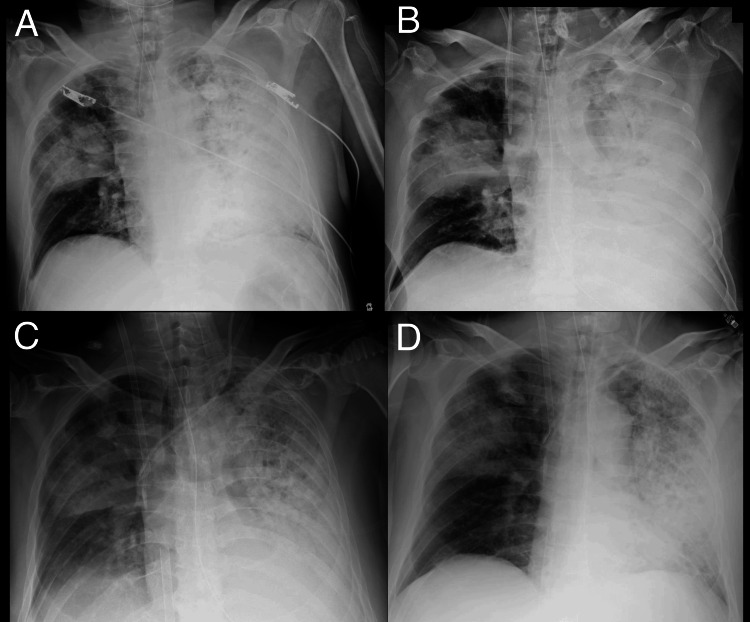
Serial chest radiographs showing the progression and recovery of ARDS in TB A chest X-ray taken upon emergency department arrival, immediately after intubation, showed reduced transparency in the right lung and diffuse opacities in the left lung (A). On the night of day 3, prior to the initiation of VV-ECMO, progressive consolidation was noted in the left lung (B). On day 9, bilateral lung opacities persist (C). On day 11, at the time of VV-ECMO removal, marked improvement in bilateral lung opacities is evident (D). ARDS: acute respiratory distress syndrome, TB: tuberculosis, VV-ECMO: venovenous extracorporeal membrane oxygenation
